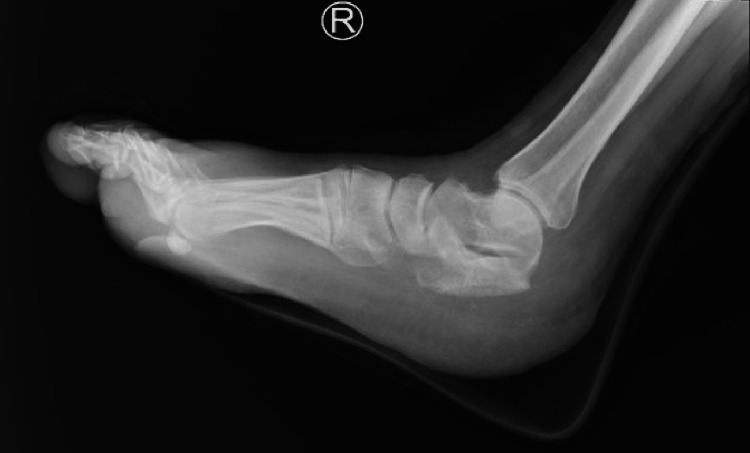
X-ray of the right foot following total calcanectomy
